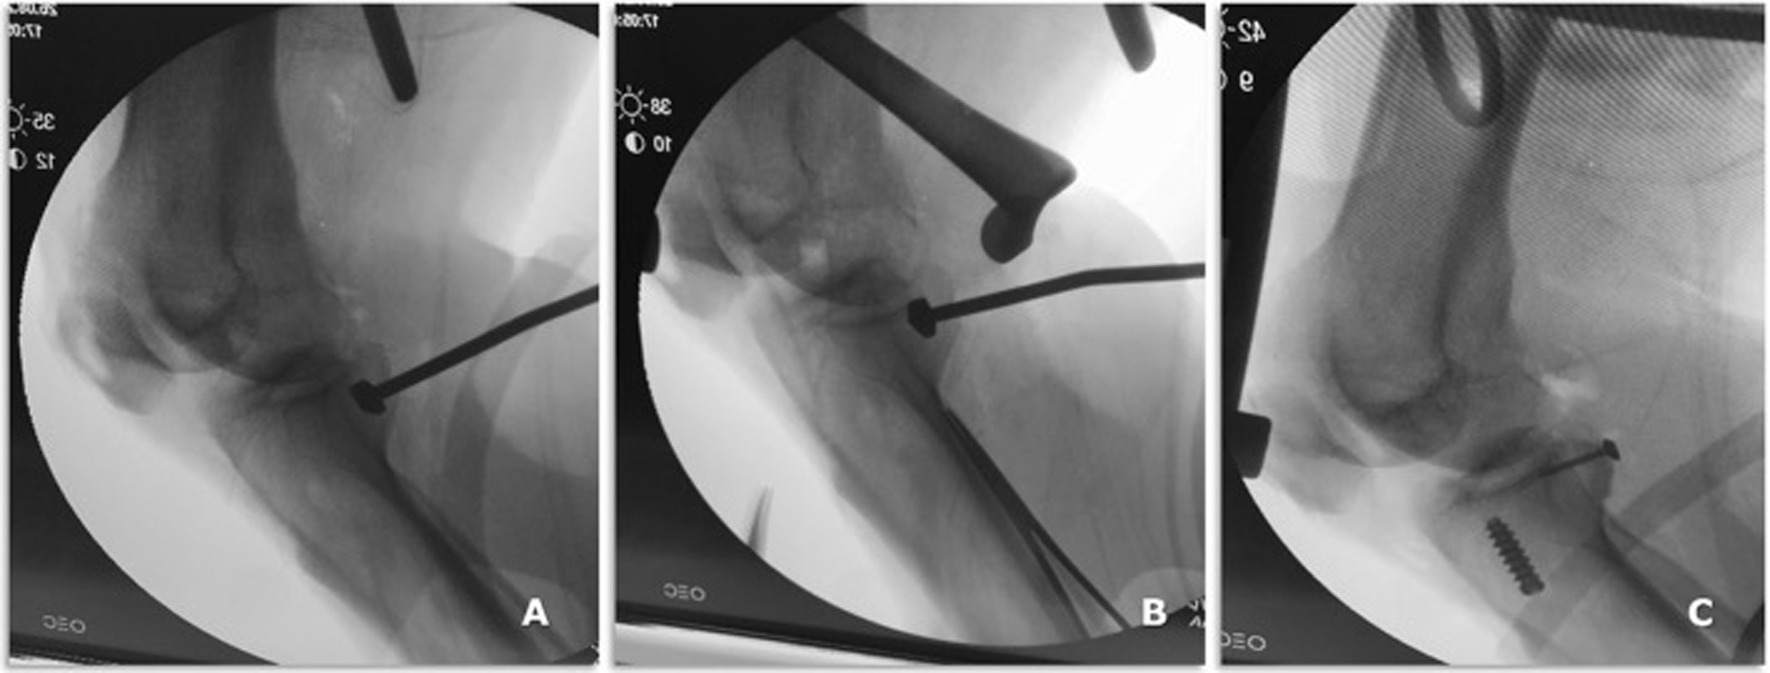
Intraoperative radiographic view of osteotome (A) and impactor (B) positioning on the PCL tibial bed and PCL and ACL graft fixation on the tibial side (C)17.Before passing the graft, a pull-out bone tunnel is added to the graft fixation by drilling a 2.5-mm tunnel just below the PCL bone slot from the posterior to the anterior aspect of the knee joint (Figs. 8A, 9)
